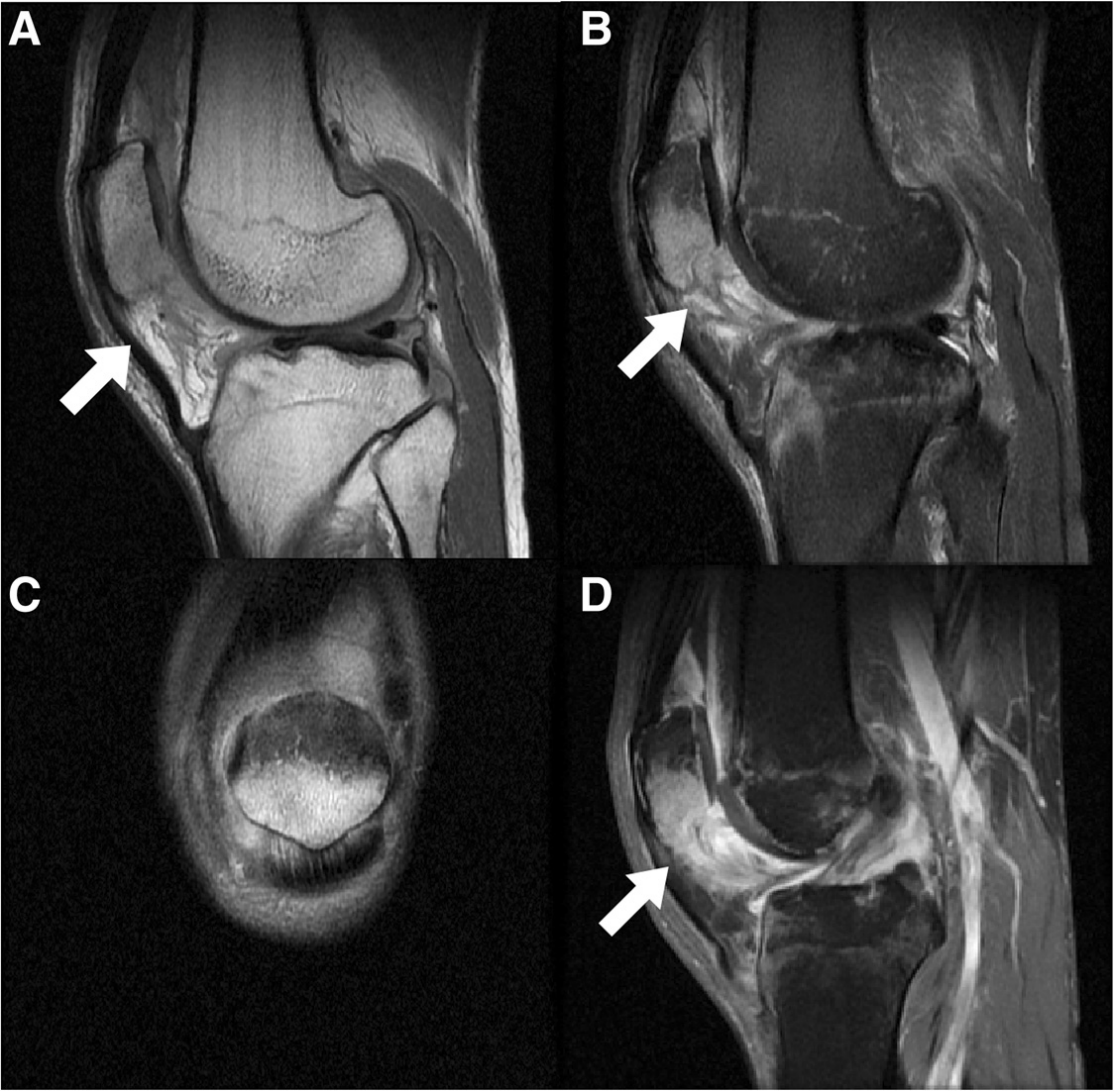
A contrasted knee MRI had been performed at an outside facility (A: Sagittal T1 proton density (TE 34; TR 1967); B: Sagittal T2 with fat saturation (TE 54; TR 3067); C: Coronal T2 with fat saturation (TE 59; TR 2733); D: Gadolinium enhanced Sagittal T1 with fat saturation (TE 10; TR 533)). This demonstrates knee joint effusion with extensive synovitis involving Hoffa’s fat (arrow: A, B, D) and continuity with the osteolytic area within the inferior pole of the patella. There is abundant adjacent bone marrow edema within the patella (B, C) and proximal tibia (B).
